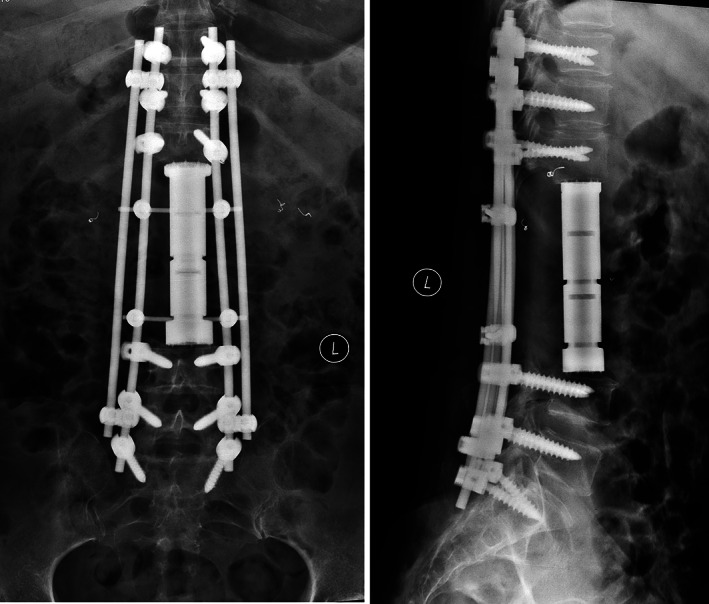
A patient (No. 21) with spinal MPNS underwent TES and reconstruction with the 3D‐printed modular vertebral prosthesis. Postoperative plain radiograph.
